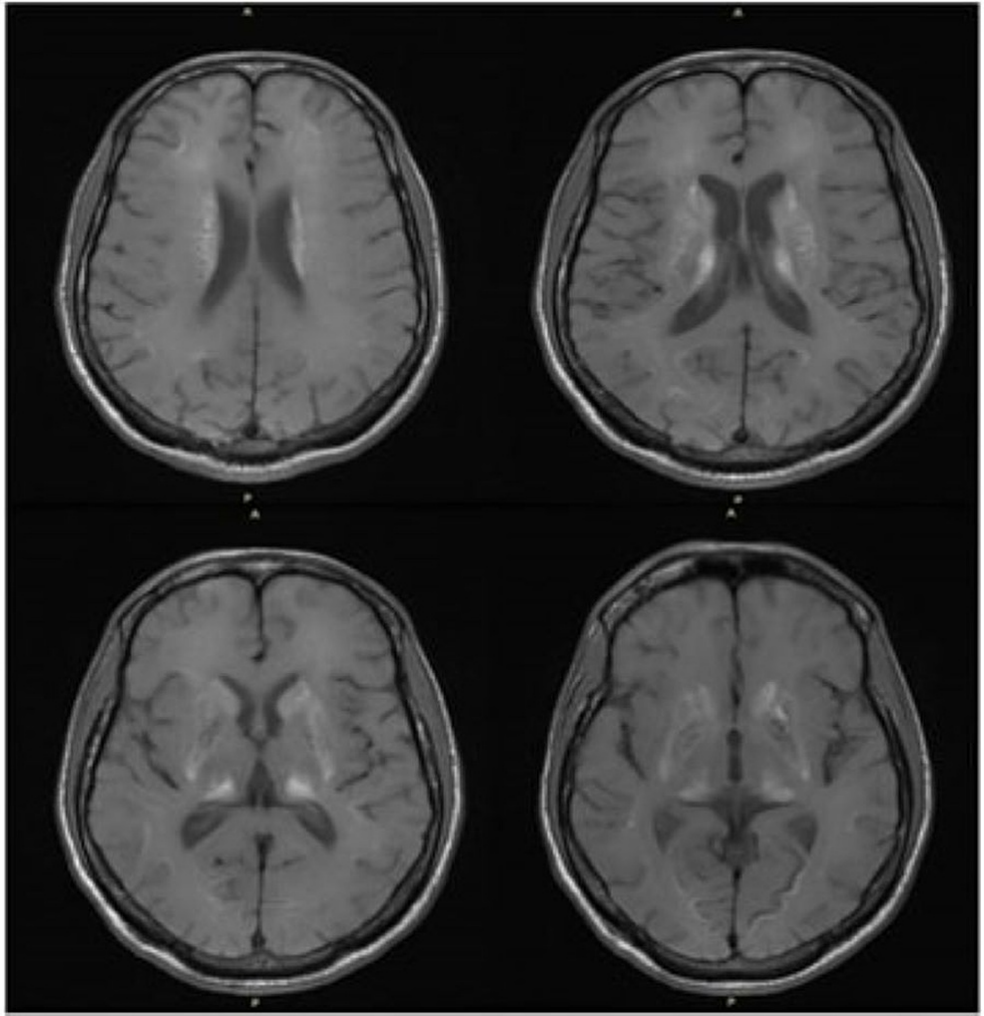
Axial MRI brain T1 hyperintensities bilateral caudate nuclei, thalami, subthalamic nuclei, and substantia nigra.
